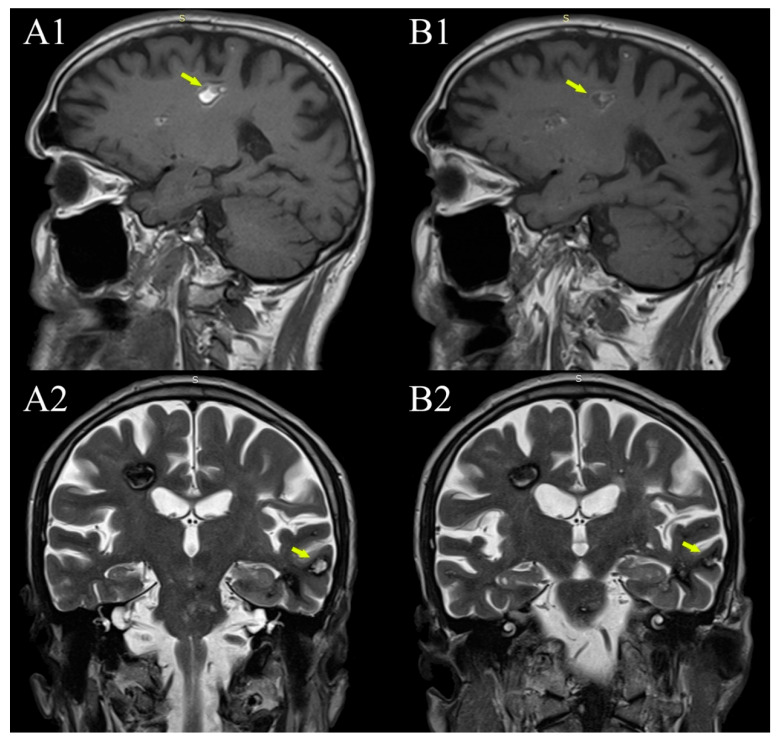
Evolution of in-cavernomas bleeding over time. (A1,A2) are from the 2015 MRI examination (1.5 T), (B1,B2) are from the 2021 examination (1.5 T). Coexistence of subacute bleeding in the right parietal lobe white matter cavernoma with T1 hyperintense signal (A1—arrow) and T2 inhomogeneous hyper- and hypointense signal and acute bleeding in a smaller left temporal lobe cavernoma with hyperintense T2 signal (A2—arrow) (but also hyperintense T1, not shown here). Both lesions became more hypointense in both T1 and T2 on the 2021 MRI examination (B1,B2 —arrows).
